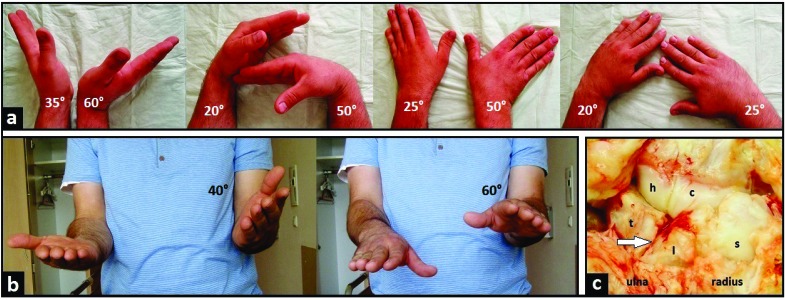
(Case Presentation, pre- and intraoperative findings): (a) Clinical photographs demonstrating impaired wrist joint motion in comparison to the uninjured right wrist; (b) Clinical photographs demonstrating impaired supination and pronation in comparison to the uninjured right wrist; (c) Intraoperative clinical photograph demonstrating posttraumatic pancarpal wrist joint OA, note the disruption of LTL (arrow) in the absence of disruption of SLL that confirmed the preoperative radiographic finding of PUCT type I.
